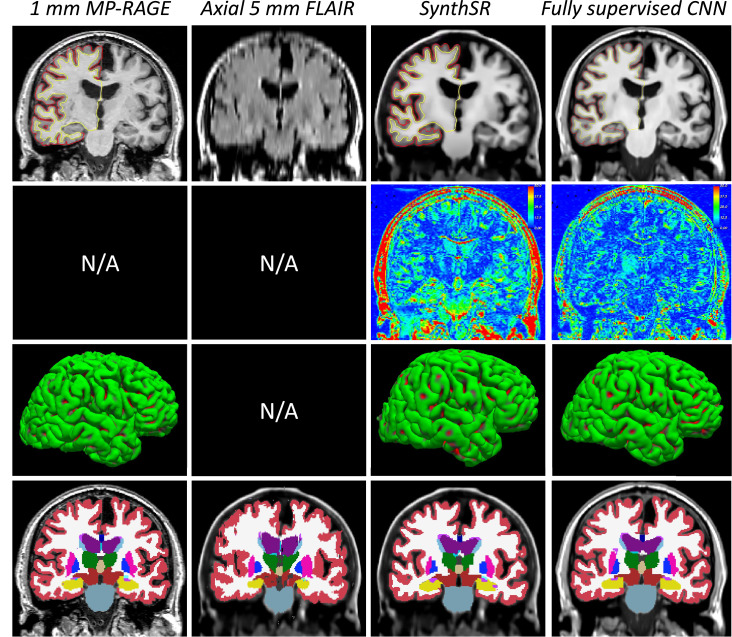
Coronal slice of a sample 1 mm T1 scan from the ADNI dataset; 5 mm axial FLAIR (with cubic interpolation); and super-resolved, with *SynthSR* and the fully supervised CNN. Top row: image intensities with pial and white matter surfaces of the right hemisphere computed with FreeSurfer 7 (not applicable to FLAIR scan). Second row: residual error maps. Third row: 3D rendering of the pial surfaces. Bottom row: volumetric segmentation obtained with FreeSurfer 7 (T1 and synthetic scans) and SAMSEG (FLAIR scan). Please note that the T1 and FLAIR scans are not perfectly aligned; we display the MP-RAGE prior to registration because resampling introduces smoothing due to interpolation artifacts (the registered scan is used to compute the residual maps).
